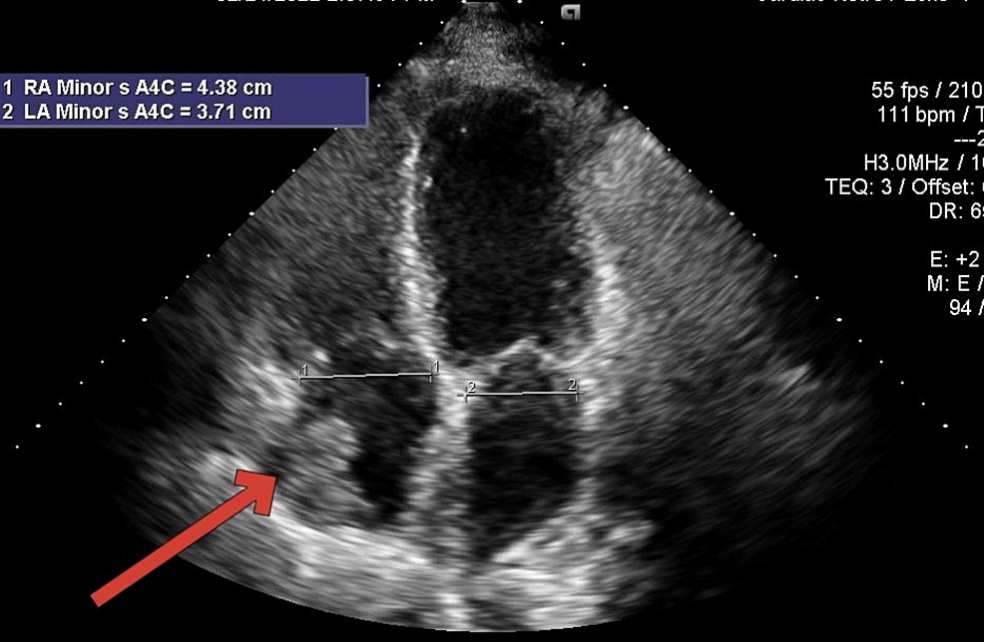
Echocardiogram in the subxiphoid view Echocardiogram in the subxiphoid view showing a red arrow pointing at a thrombus in the right atrium
